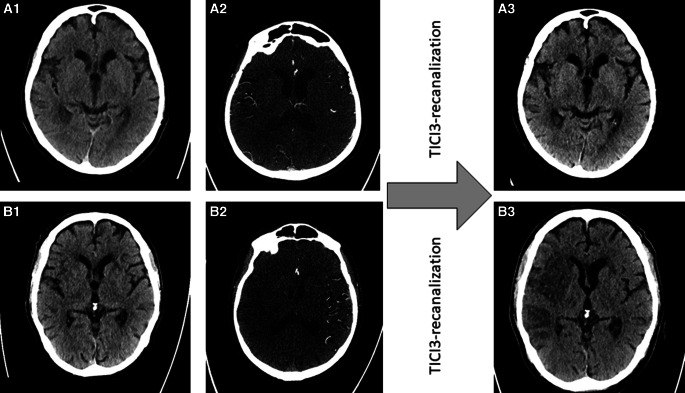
Acute and follow-up imaging of two exemplary patients (A and B) who both underwent acute CT imaging within less than 100 min after symptom onset and who both underwent complete recanalization (mTICI3) of their right-sided middle cerebral artery M1 segment occlusion (patient A within 30 min, patient B within 39 min after image acquisition). On acute NCCT (A1 + B1), early ischemic changes were found for both patients along the right insular ribbon. However, patient A had excellent collateral supply on CT-angiography (A2), while patient B had no collateral filling (B2). On follow-up imaging, large infarction is observed in patient B (B3), while ischemic changes are still confined to the insular ribbon in patient A (A3)
